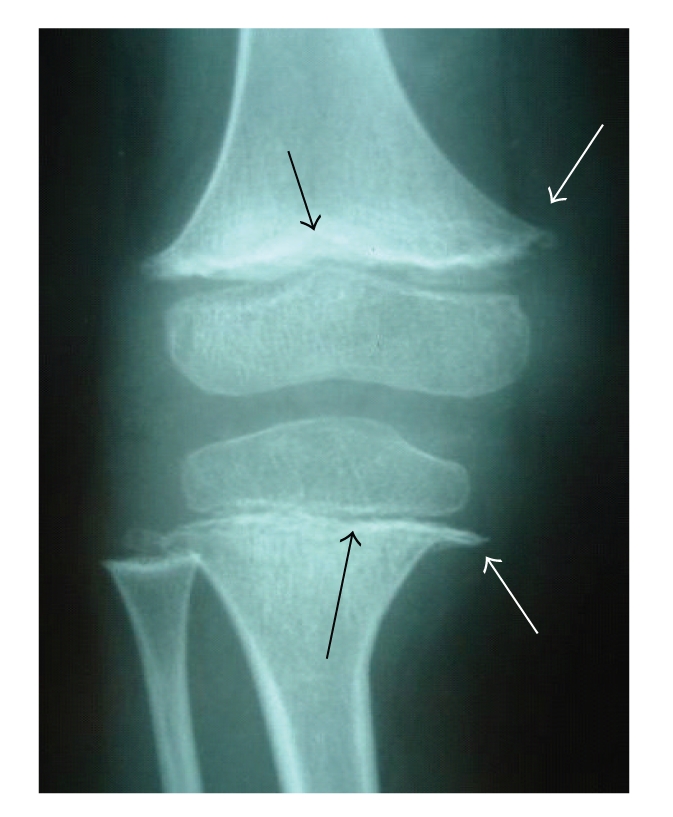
Anterior-posterior radiograph of the knee showing osteopenia, a thickened white line at the metaphysis of both femurs and tibiae (black arrow) and a small beak-like excrescences at the metaphysic of femur and tibi (white arrows).
